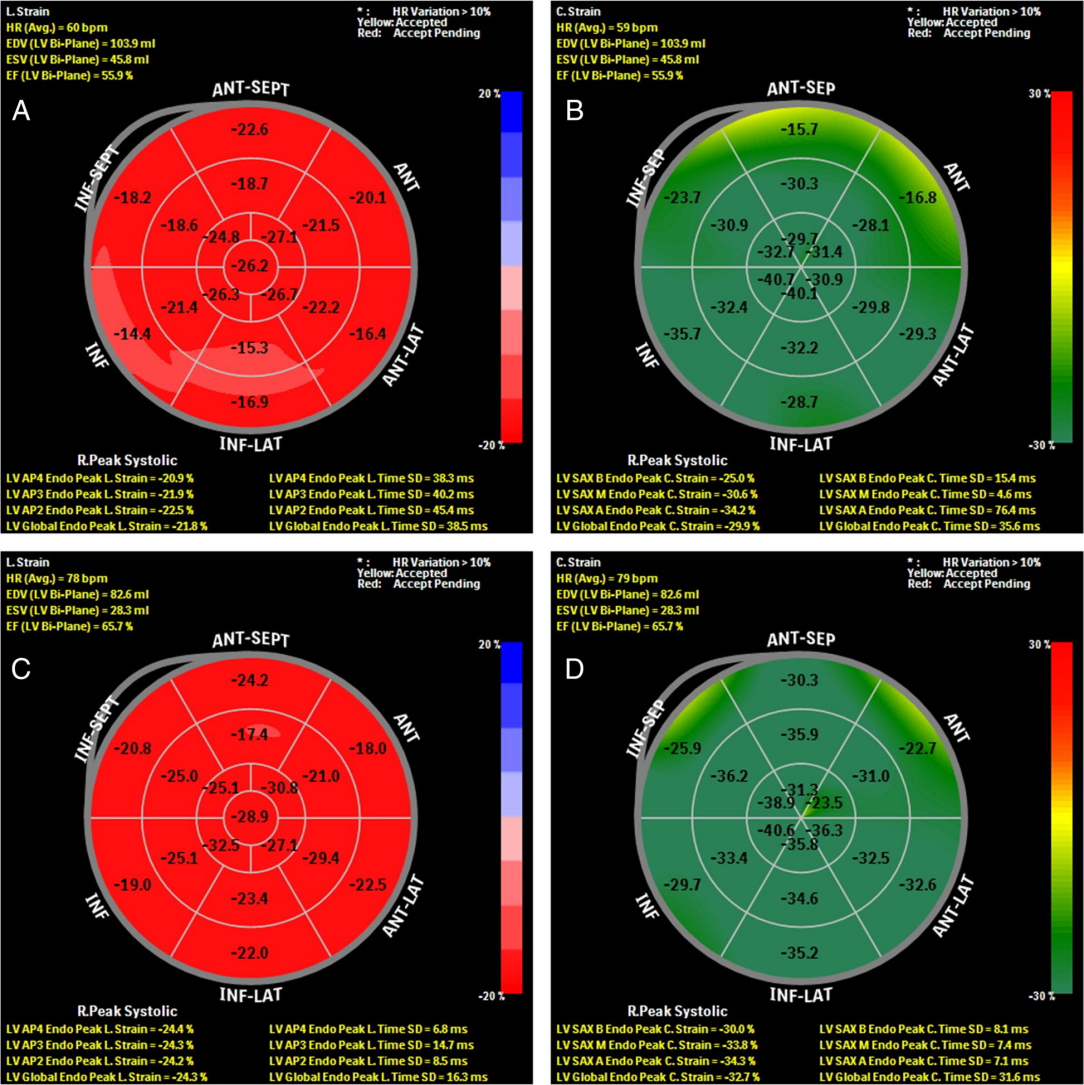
Example of assessment of LV endocardial LS and CS by speckle-tracking echocardiography in patients with SLE (A: LV endocardial LS = -21.8%, B: LV endocardial CS = -29.9%) compared with healthy control (C: LV endocardial LS = -24.3%, D; LV endocardial CS = -32.7%) individual displayed with red-blue-, and green–red-coded bull’s-eye plots for LS and CS
